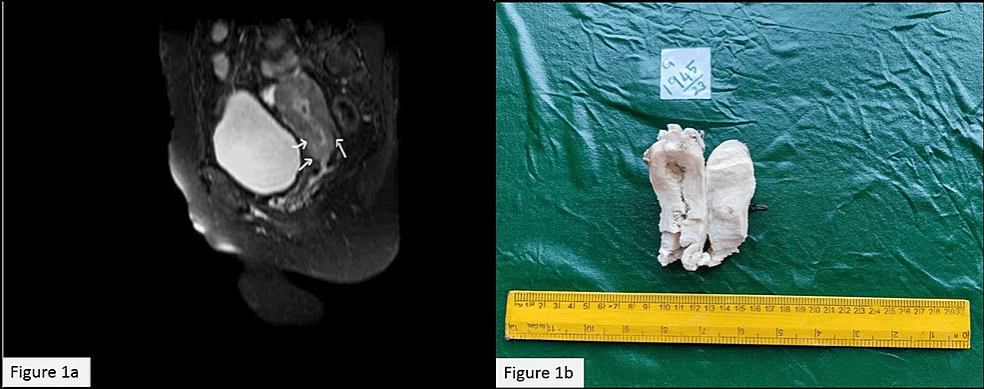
(a) MRI T2 weighted image: axial view of an ill-defined lesion in the endocervical canal extending superficially into the endometrium. (b) Gross appearance of the tumor extending from the cervix into the endometrium MRI: magnetic resonance imaging
